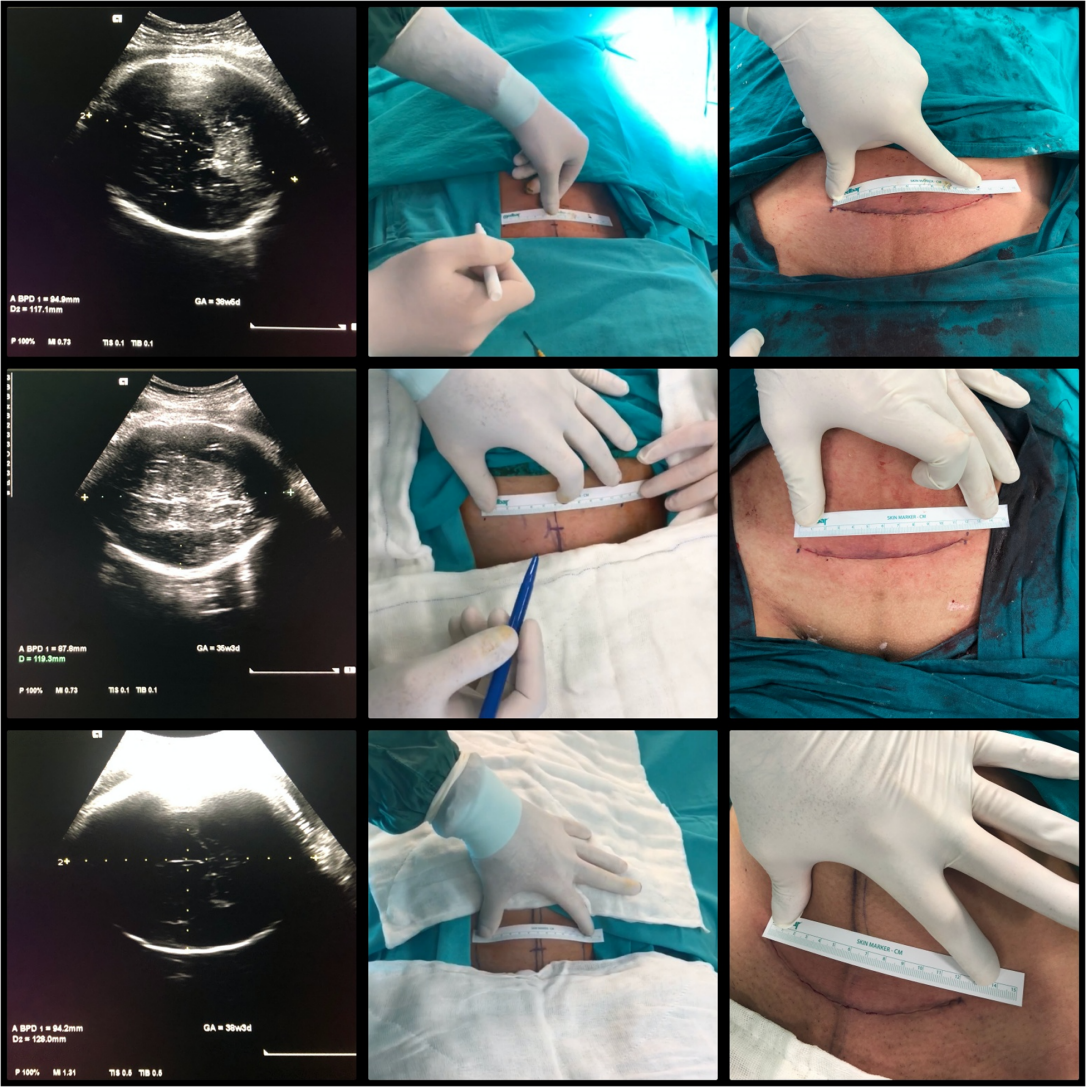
Adjusting the Pfannenstiel skin incision according to the ultrasound measurement of occiputofrontal diameter (OFD). (Pfannenstiel incision with Kaya modification). OFD's were measured as 117, 119 and 128 mm from top to bottom, respectively. Pfannenstiel incisions after closure of the skin were measured as 128, 117 and 125 mm from top to bottom, respectively.
